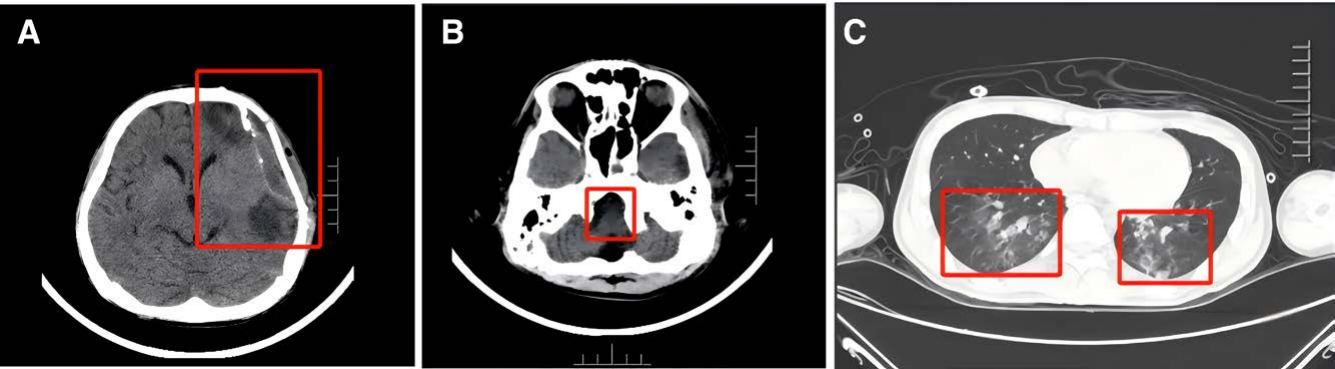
(A and B) CT of the brain demonstrated low-density lesions in the medulla and left frontotemporoparietal region. (C) CT of chest showed multiple inflammatory patchy shadows in both lungs.
